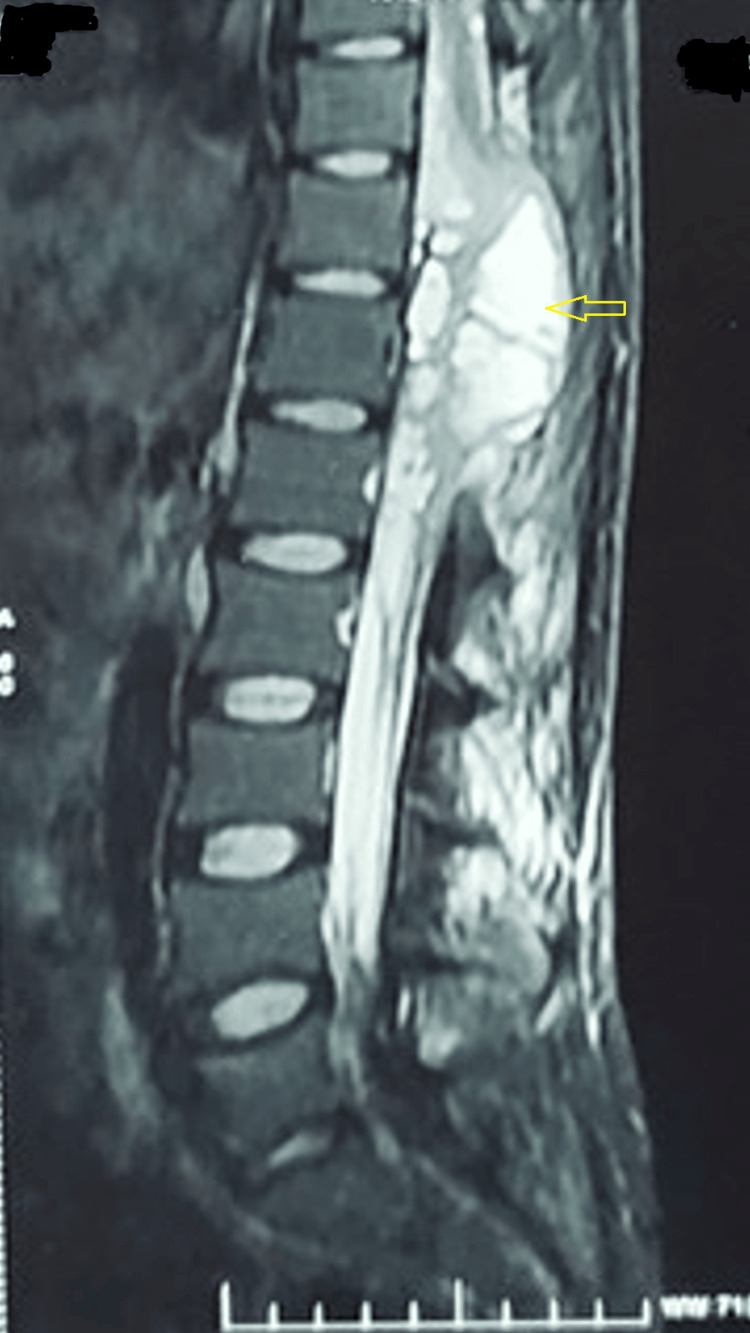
An MRI of the thoracolumbar spine (mid-sagittal image) Loculated fluid collection with contrast enhancement in the spinal canal extending from T9 to T12 (yellow arrow) vertebral levels involving the distal cord, conus medullaris, and cauda equina nerve roots
